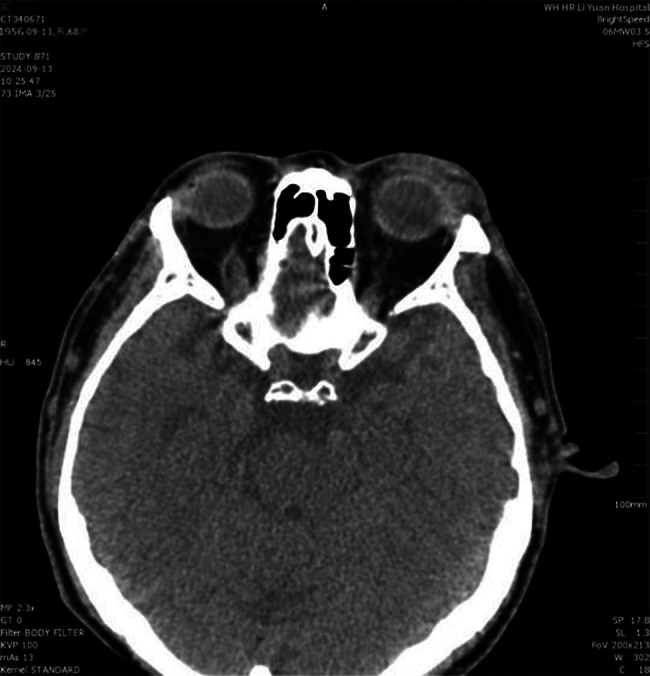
Axial CT scan revealed a well-circumscribed, nodular soft-tissue density lesion in the lateral aspect of the orbit, adjacent to the orbital lobe of the lacrimal gland
